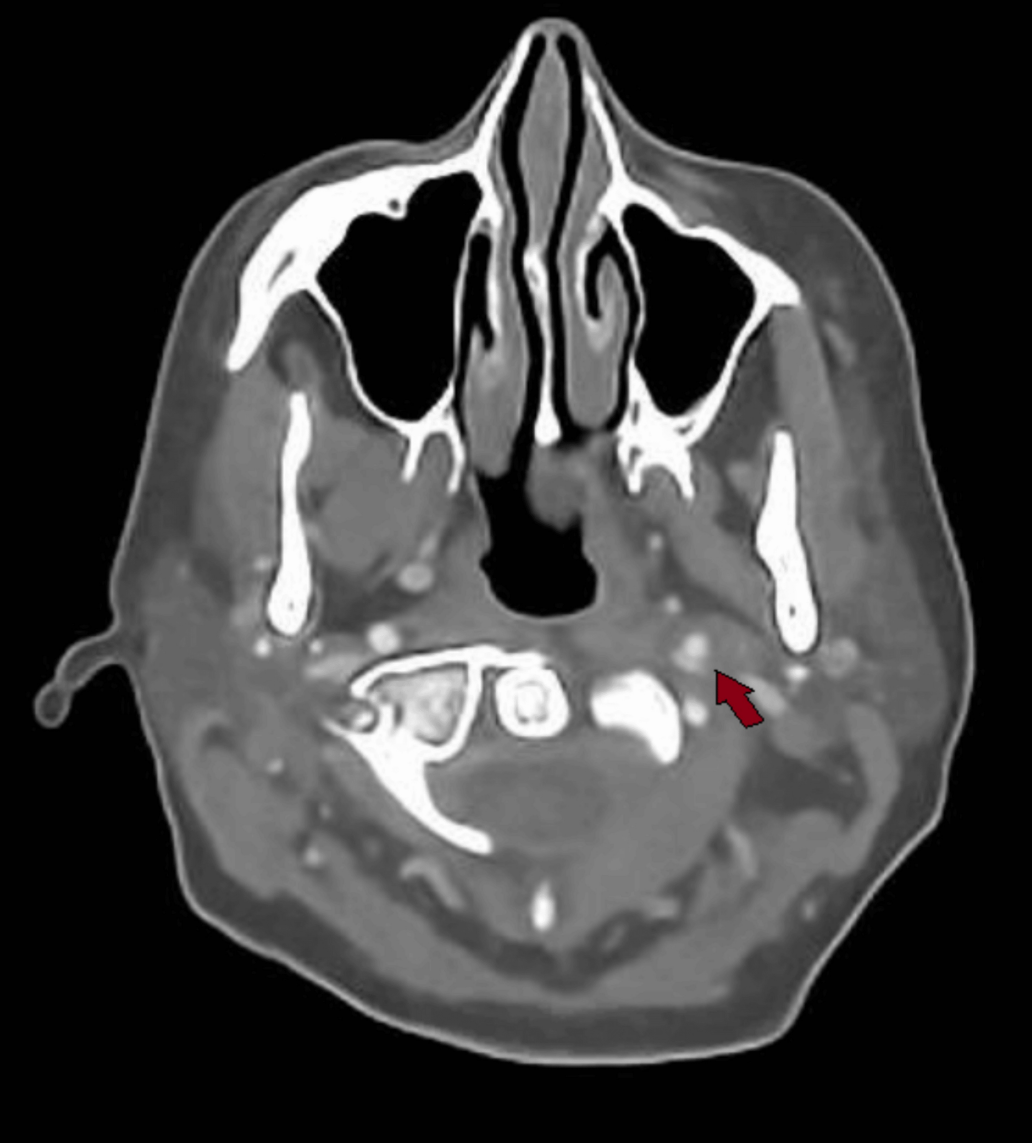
Axial CT carotid angiogram demonstrating a left extracranial internal carotid artery dissection flap with a false lumen.
